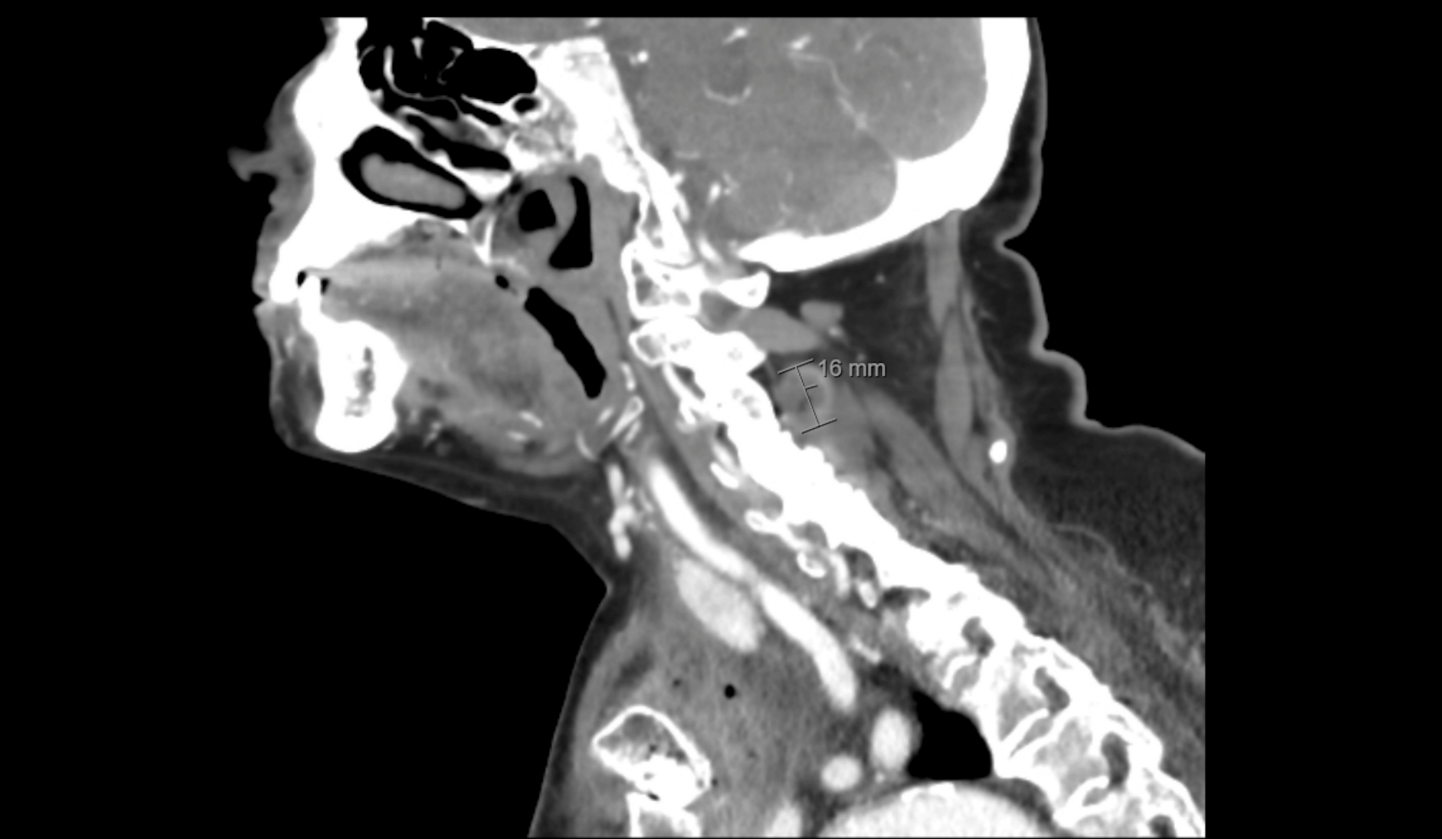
Posterior cervical paravertebral abscess at the C4–C5 level on sagittal computed tomography Sagittal computed tomography of the cervical spine demonstrating a posterior cervical paravertebral abscess at the C4–C5 level, measuring approximately 16 millimetres in depth, associated with surrounding inflammatory changes of the posterior elements.
